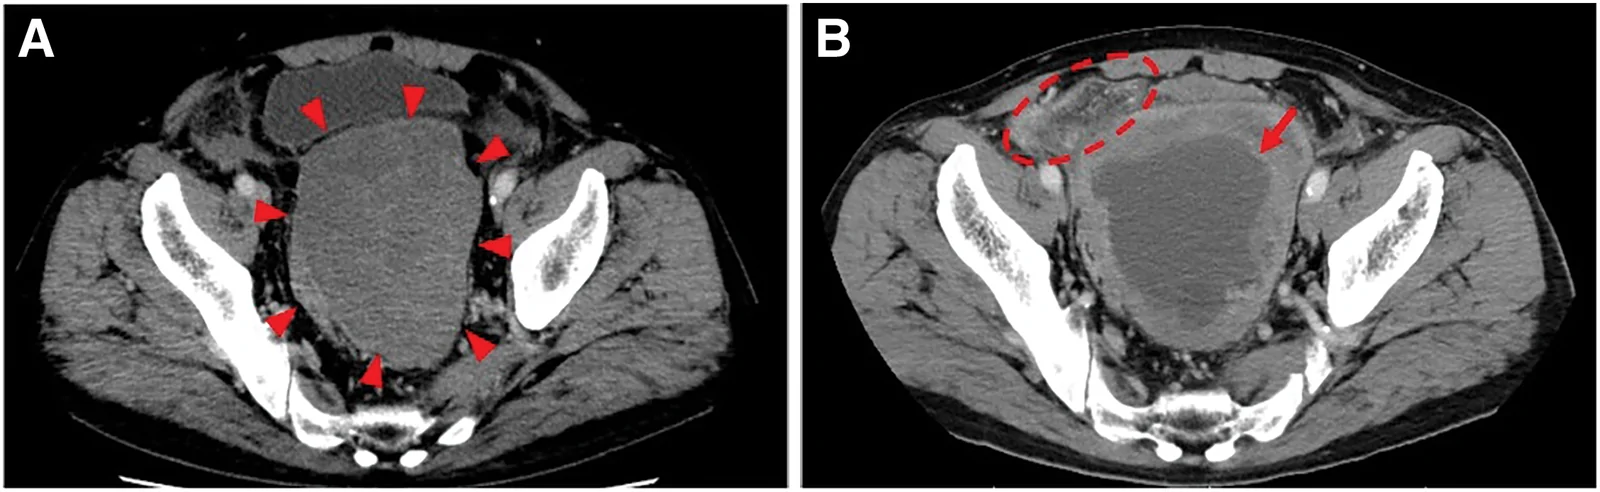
CT findings. (A) Preoperative contrast-enhanced CT obtained at the previous hospital 5 weeks ago showed a well-defined heterogeneous pelvic mass measuring 12 × 9 cm with poor enhancement (arrowheads) and associated ascites. (B) Emergency CT obtained 1 week later showed slight enlargement of the tumor, newly developed intratumoral fluid collection (arrow), and increased fat stranding around the tumor (dotted circle).
